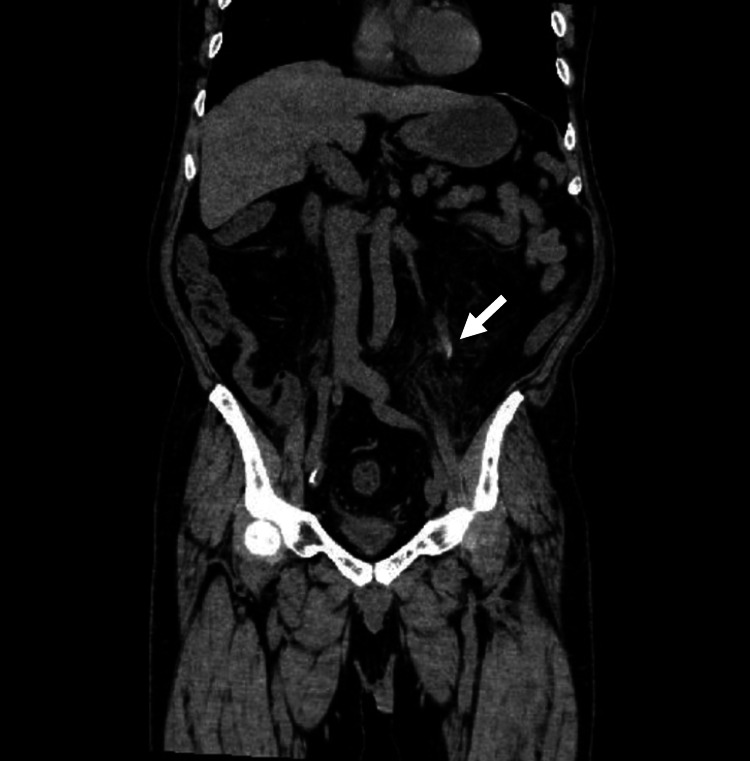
A coronal plane of computed tomography Computed tomography showed hematoma within the extensive ureter (white arrow).
